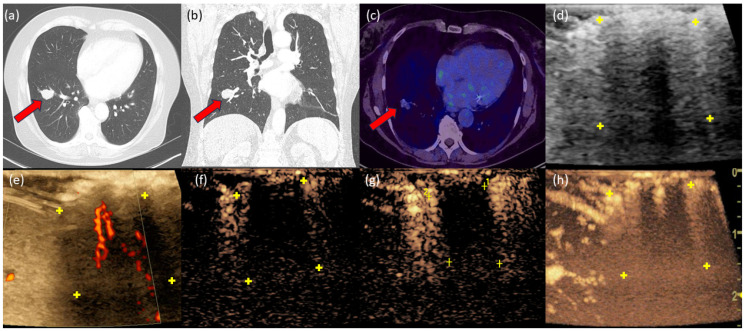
In the CT thorax, a clearly partially lobulated SPN can be delineated in the right lower lung lobe (a,b). The red arrow marks the tumor. In the PET-CT (c), the SPN appears faintly. In B-mode (d), a partially hypo- and partially hyperechoic structure can be delineated. (e). The yellow “+” marks the tumor margin. The power Doppler with peripheral and central vessel sections. In CEUS, an early peripheral contrast enhancement is observed in the wash-in phase at t = 12 s (f), which intensifies over time at t = 19 s (g). The 3D reconstruction shows central contrast agent uptake with a large void (h).
